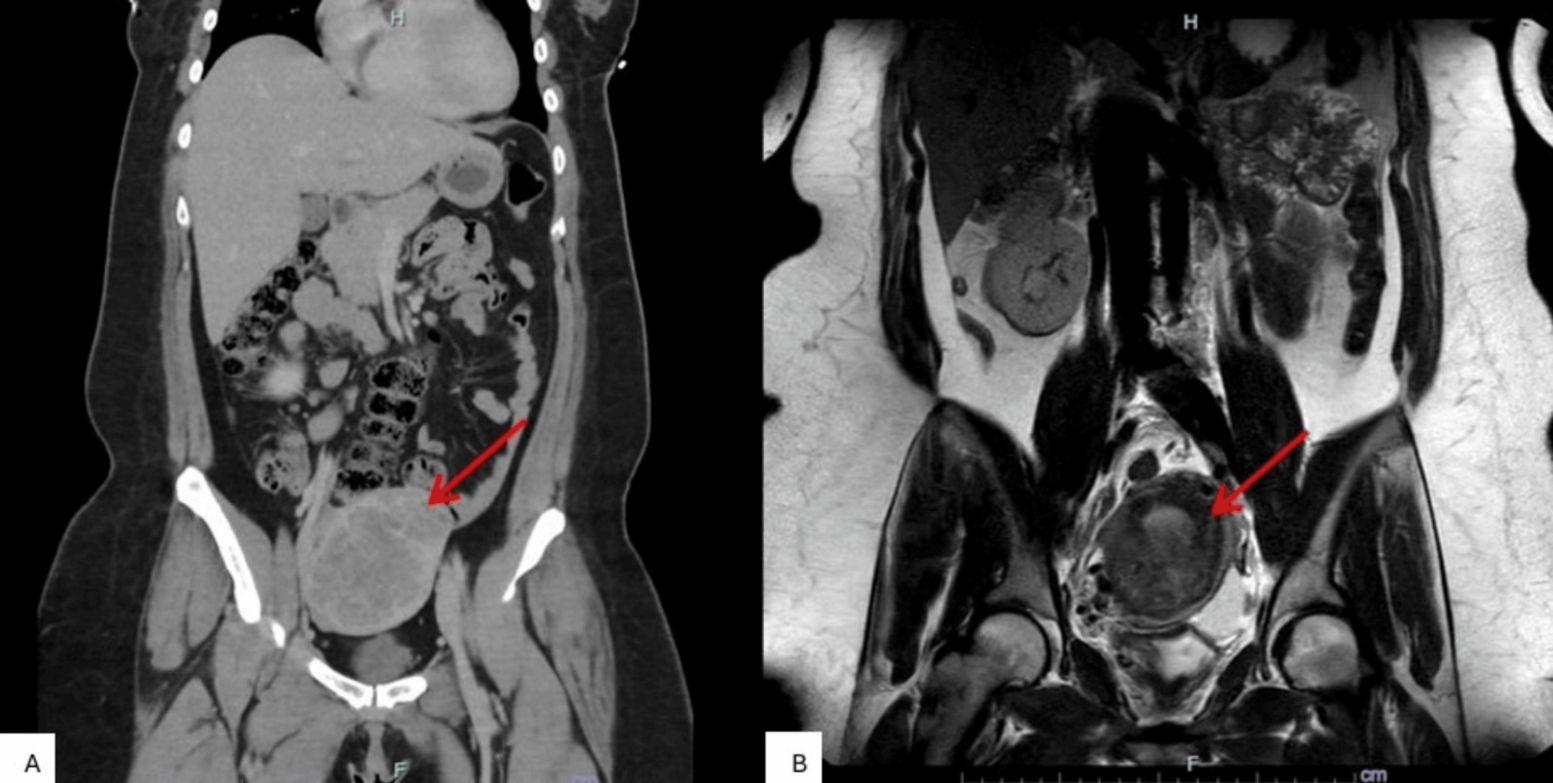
Cross-sectional imaging demonstrating a pelvic organ tumor. A. Coronal contrast-enhanced computed tomography of the abdomen and pelvis demonstrating a large, well-circumscribed heterogeneous mass within the pelvis (red arrow), extending into the lower abdomen. B. Coronal T2-weighted magnetic resonance imaging of the pelvis demonstrating the same lesion (red arrow) with heterogeneous signal intensity, providing improved delineation of the pelvic origin.
